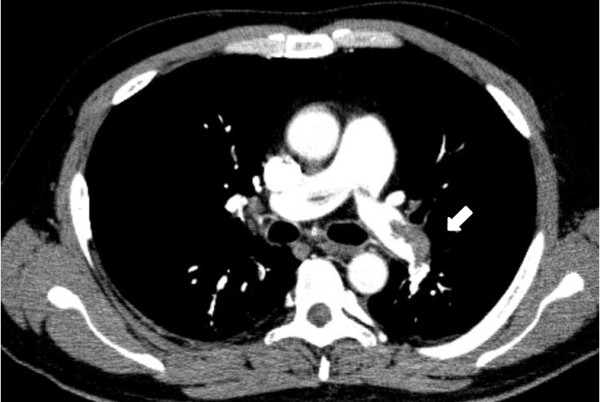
CT scan showing submassive PE.
